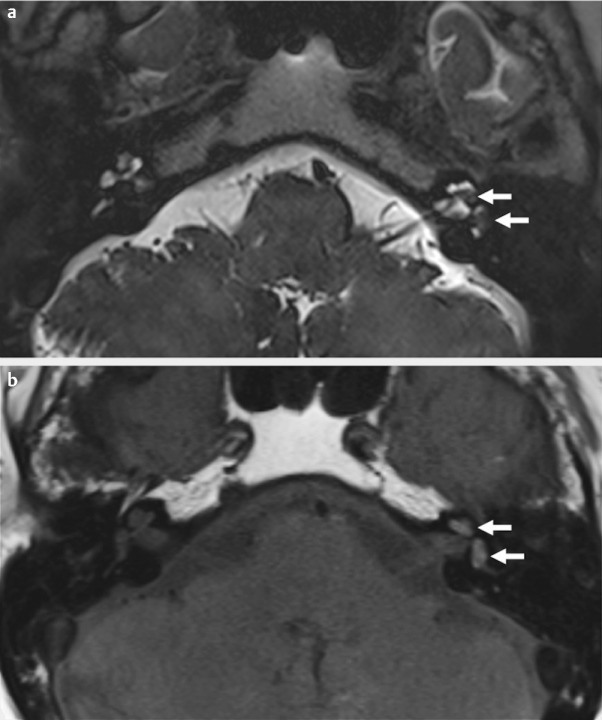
Spontaneous labyrinthine bleeding in the left inner ear. Acute left-sided deafness and vertigo of a patient after therapy for Non-Hodgkin-lymphoma of the central nervous system (Ann Arbor stage IV) 3 years before and drug-induced coagulopathy (anticoagulation medication) due to port thrombosis. In the area of the cochlear as well as the vestibular part of the inner ear, MRI revealed punctually clear signal decreases (arrows) in the T2-weighted (a) and a minor signal increase (arrows) in the native T1-weighted images (b). w: weighted. Department of Radiology, University Medicine Halle, courtesy of Prof. S. Kösling.
